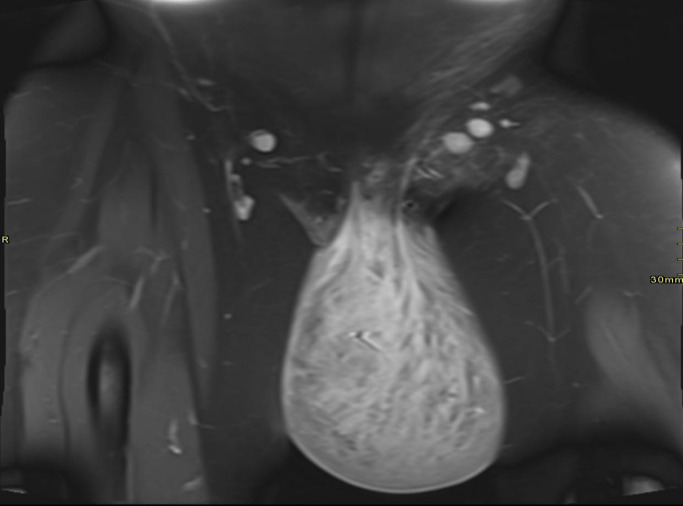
Coronal T2-weighted MRI demonstrates the pendulous configuration of the vulvar mass extending inferiorly from the left vulva.
